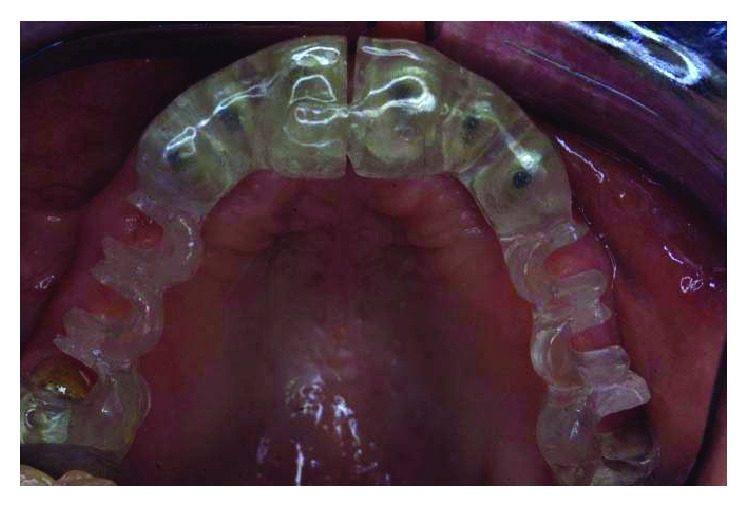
Surgical stents were fabricated based upon the information from the CT scan and using the diagnostic wax-up.
